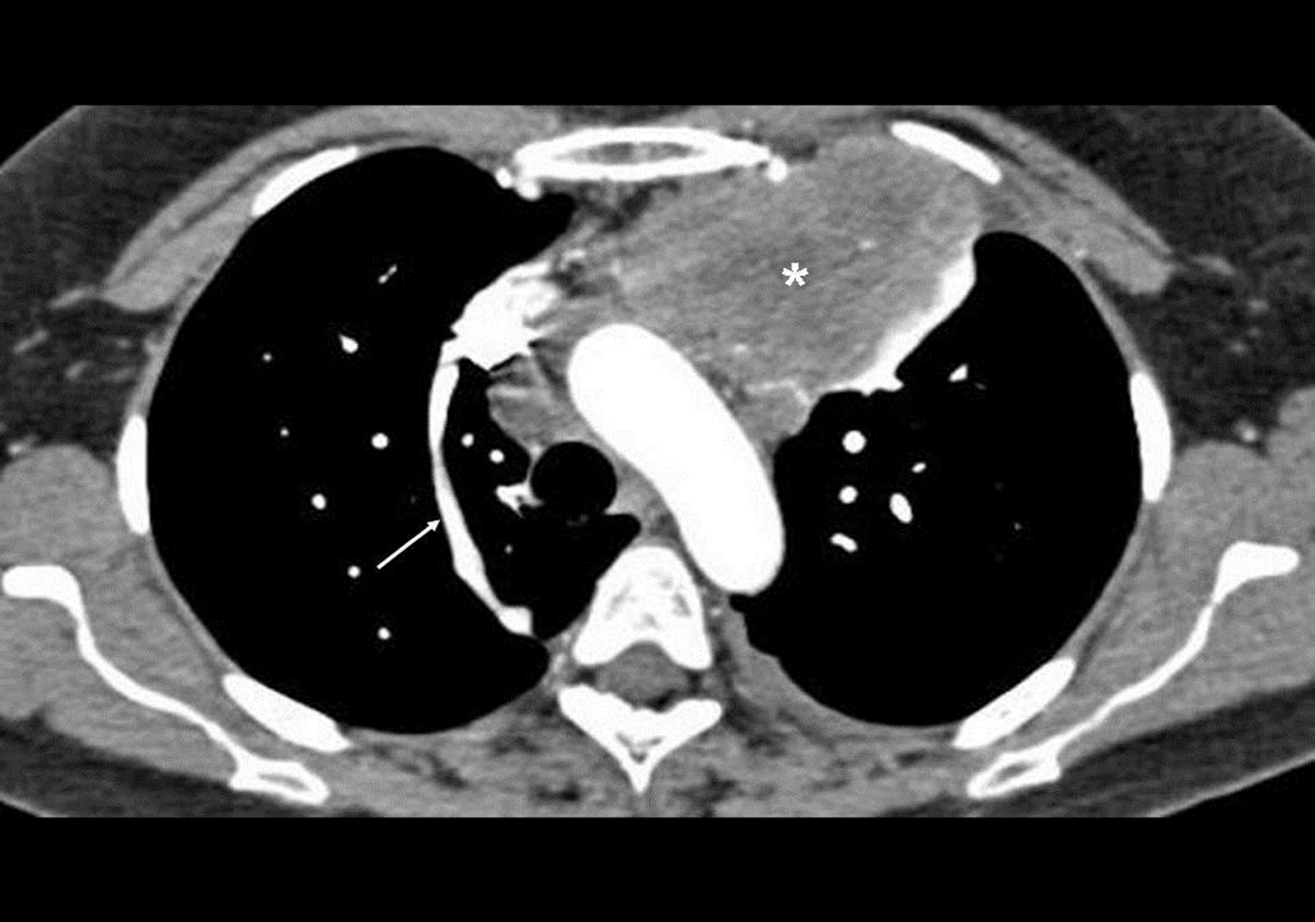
Contrast-enhanced CT of the chest of an 18-year-old shows a large anterior mediastinal mass (asterisk), which was subsequently diagnosed to be a germ cell tumour. The linear vascular structure (arrow) entering the posterior aspect of the superior vena cava is the azygous vein. It is to be noted that the appearance of azygous vein can vary and is dependent not only on its course, but also on the direction of the X-ray beam.
